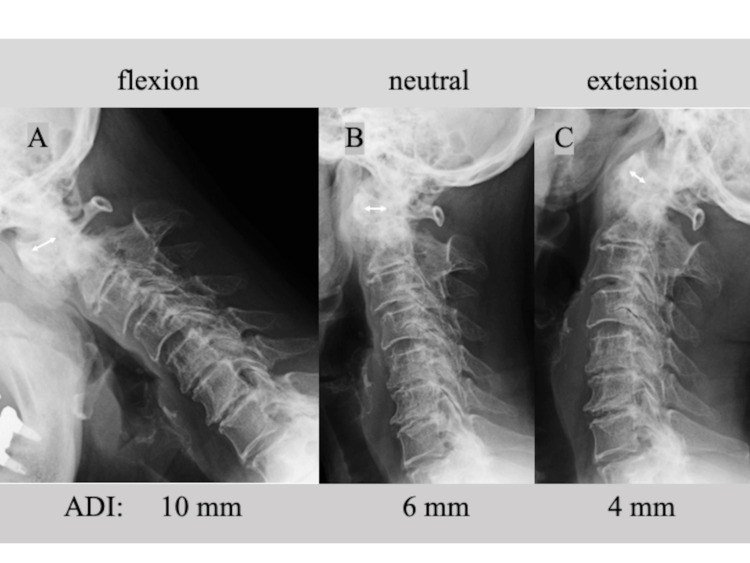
Lateral cervical spine plain X-rays at initial presentation showing an increase in the atolantodental interval of (A) 10 mm in flexion, (B) 6 mm in neutral, and (C) 4 mm in extension (white double-headed arrows). ADI: atlantodental interval
